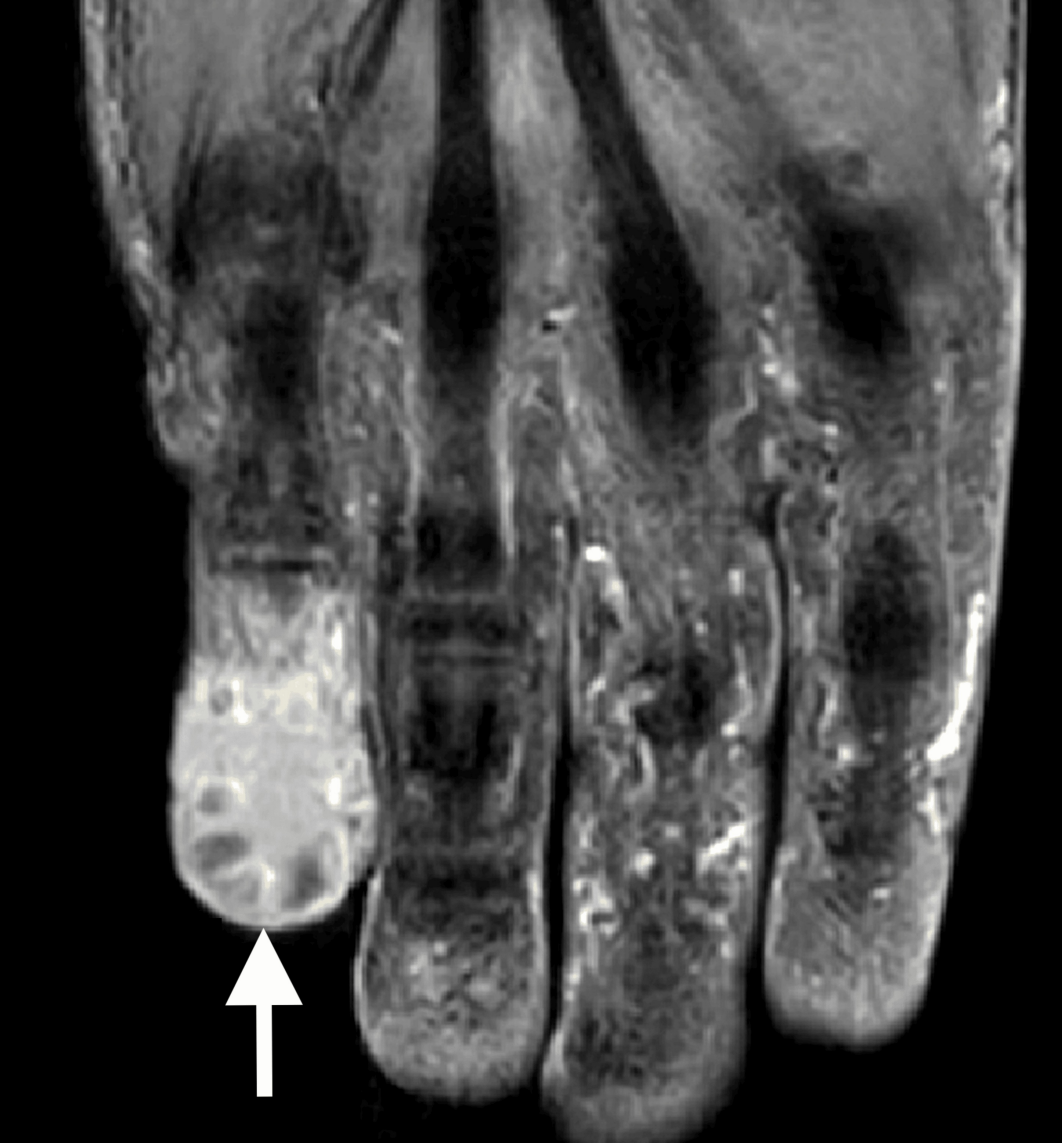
MRI of the right hand with and without gadolinium. Imaging demonstrates an expansile lytic lesion in the distal phalanx of the small finger with involvement of the middle phalanx (arrow).
